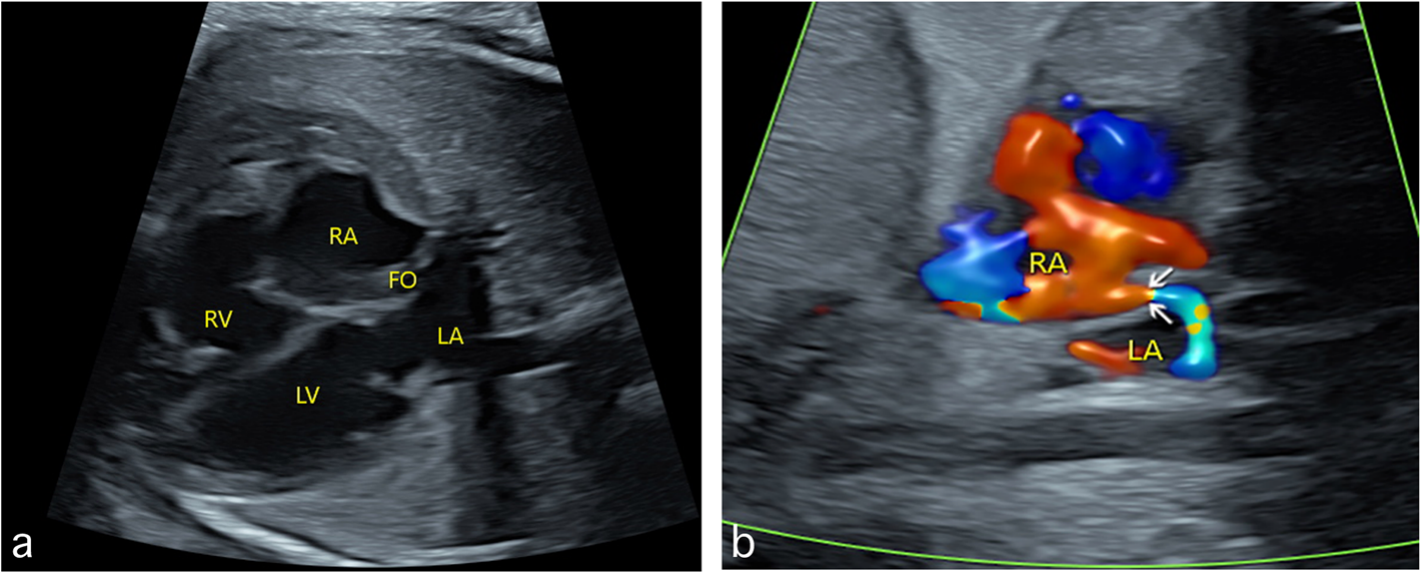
Sonograms showing fetal restrictive foramen ovale. RA dilatation is shown in the four-chamber view (a). A sagittal view clearly shows the small gap (indicated by the arrows) between a fixed FO valves and the septum secondum, which is the real entrance of FO to the LA (b). FO: foramen ovale; LA: left atrium; LV: left ventricle; RA: right atrium; RV: right ventricle
